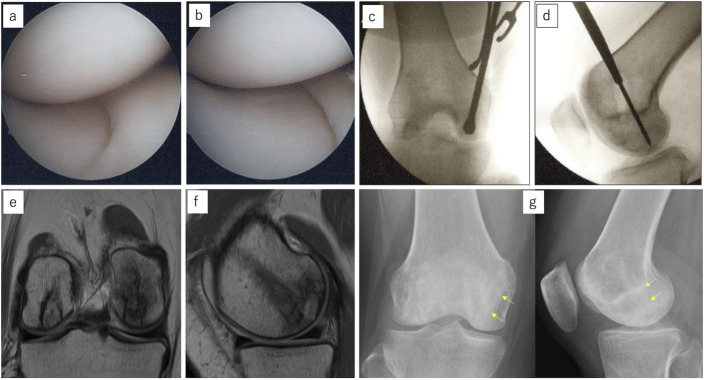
Intraoperative and postoperative findings of the case (Patient No. 7, right knee). Fig. 3-A Arthroscopic view at 90° of knee flexion obtained before curettage, demonstrating an intact articular cartilage surface without fissuring or delamination. Fig. 3-B Arthroscopic view in deep knee flexion obtained before curettage, confirming preservation of the cartilage surface in the posterior aspect of the medial femoral condyle. Figs. 3-C and 3-D In this case, drilling toward the necrotic lesion was performed using a cannulated drill under fluoroscopic guidance to facilitate access to the subchondral region, followed by use of an expandable reamer (9-mm diameter) to further evacuate necrotic bone, and subsequent curettage. Arthroscopic evaluation was performed after debridement to confirm that no iatrogenic damage to the articular surface had occurred. Figs. 3-E and 3-F Postoperative proton density-weighted magnetic resonance imaging obtained 6 months after surgery showing preservation of the articular surface without evidence of subchondral collapse. Fig. 3-G Plain radiograph obtained at the final follow-up (4 years postoperatively) demonstrating graft incorporation (yellow arrows) without progression of subchondral collapse. On this final follow-up radiograph, the untreated lateral femoral condylar lesion, which was considered asymptomatic preoperatively, showed no obvious radiographic progression or subchondral collapse during the 4-year follow-up period.
